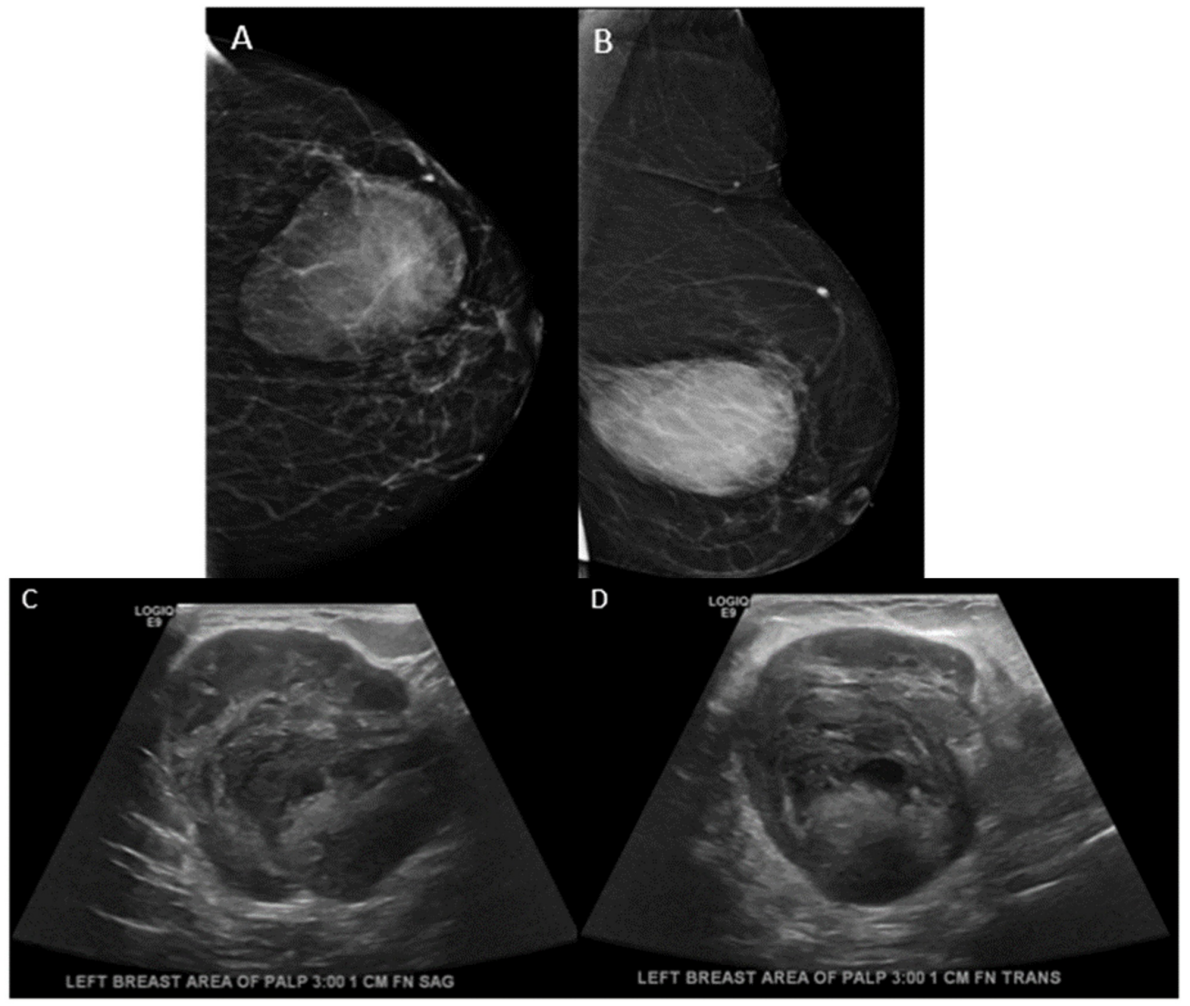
Case 5 initial imaging findings A, B: Craniocaudal and mediolateral oblique tomosynthesis views of the left breast show an oval, high-density mass with circumscribed margins in the central outer left breast corresponding to the area of palpable concern. C, D: Ultrasound grayscale imaging demonstrates a round heterogeneous mass with small central cystic areas and partially circumscribed margins.
